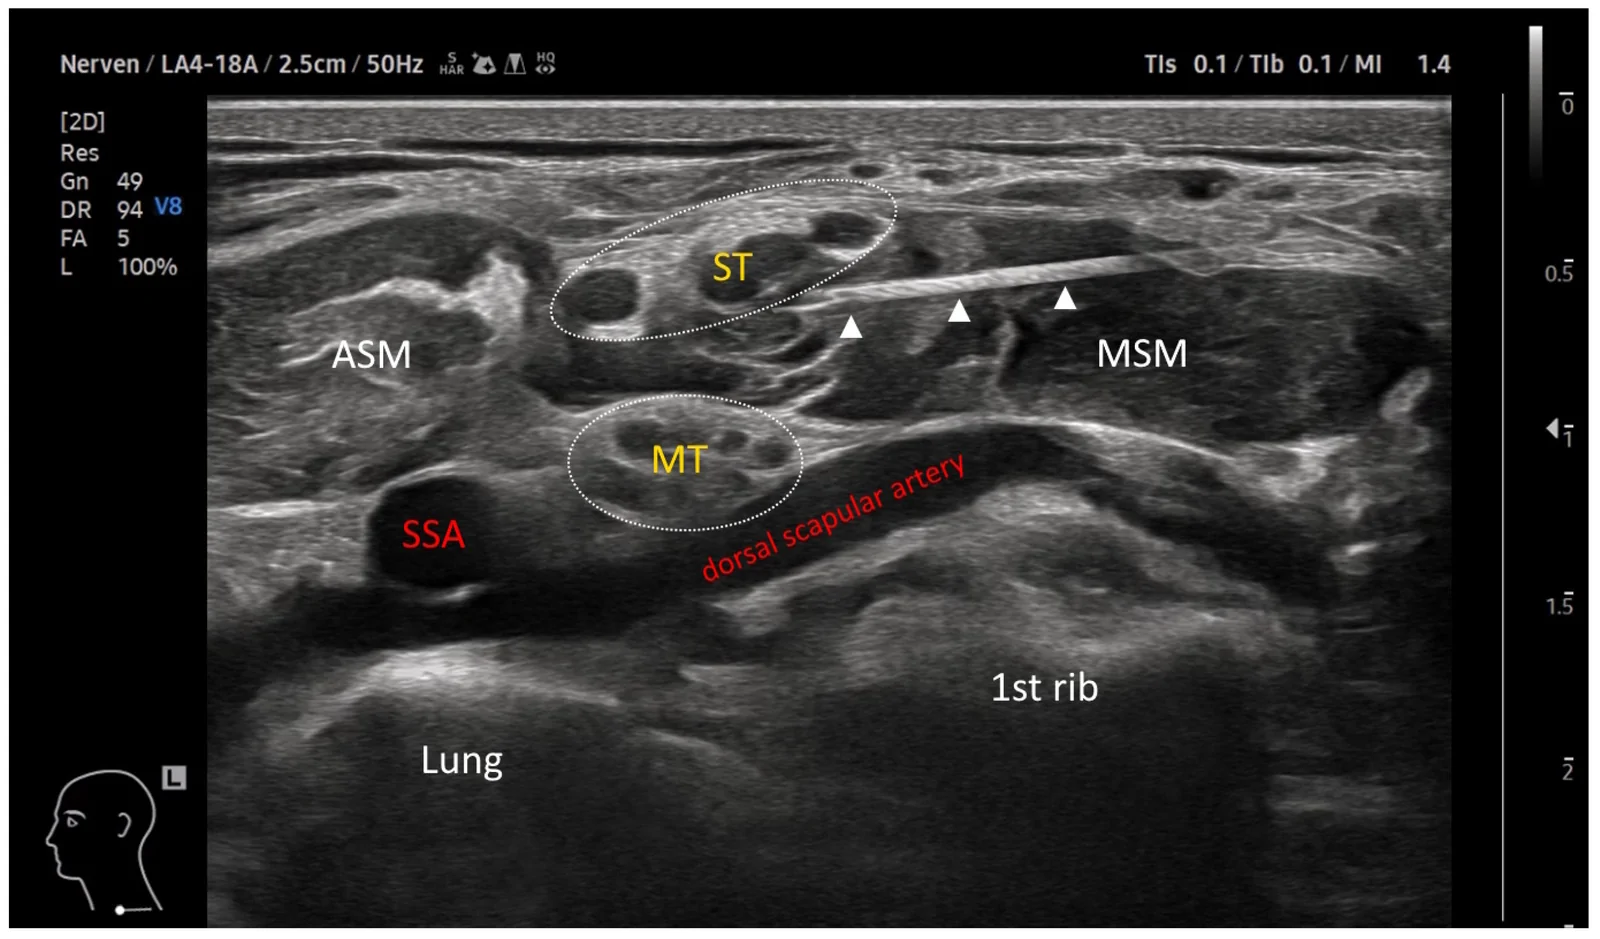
Supraclavicular approach to the superior trunk. Ultrasound image in the supraclavicular region demonstrating the superior trunk (ST) as the primary target. The middle trunk (MT), suprascapular artery (SSA), and first rib are visible. The pleura/lung is seen deep to the rib. Compared to the interscalene level, the phrenic nerve is typically located more medially and farther away from the ST. The injection needle with its visible tip is indicated by white arrows.
